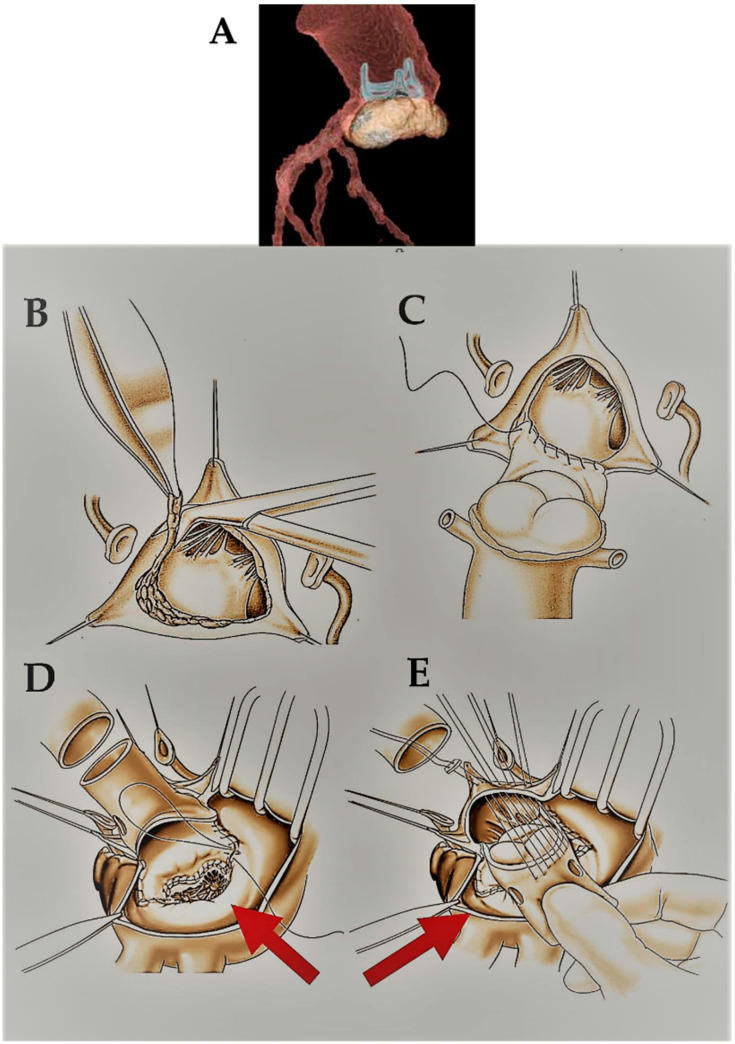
(A) Three-dimensional CT Scan reconstruction of PVE with extended periannular abscess. (B,C) A homograft is used for aortic root reconstruction and for the repair of mitro-aortic curtain using the miniroot procedure. The infected prosthesis is removed with aggressive debridement of all infected and necrotic tissue. The coronary ostia are prepared for the reconstruction of the aortic root. (D,E) Aortic and mitral homograft. Mitro-aortic endocarditis with the aortomitral curtain was largely involved. The abscess cavity is precisely bounded and debrided. A double homograft was used for the reconstruction (red arrows).
